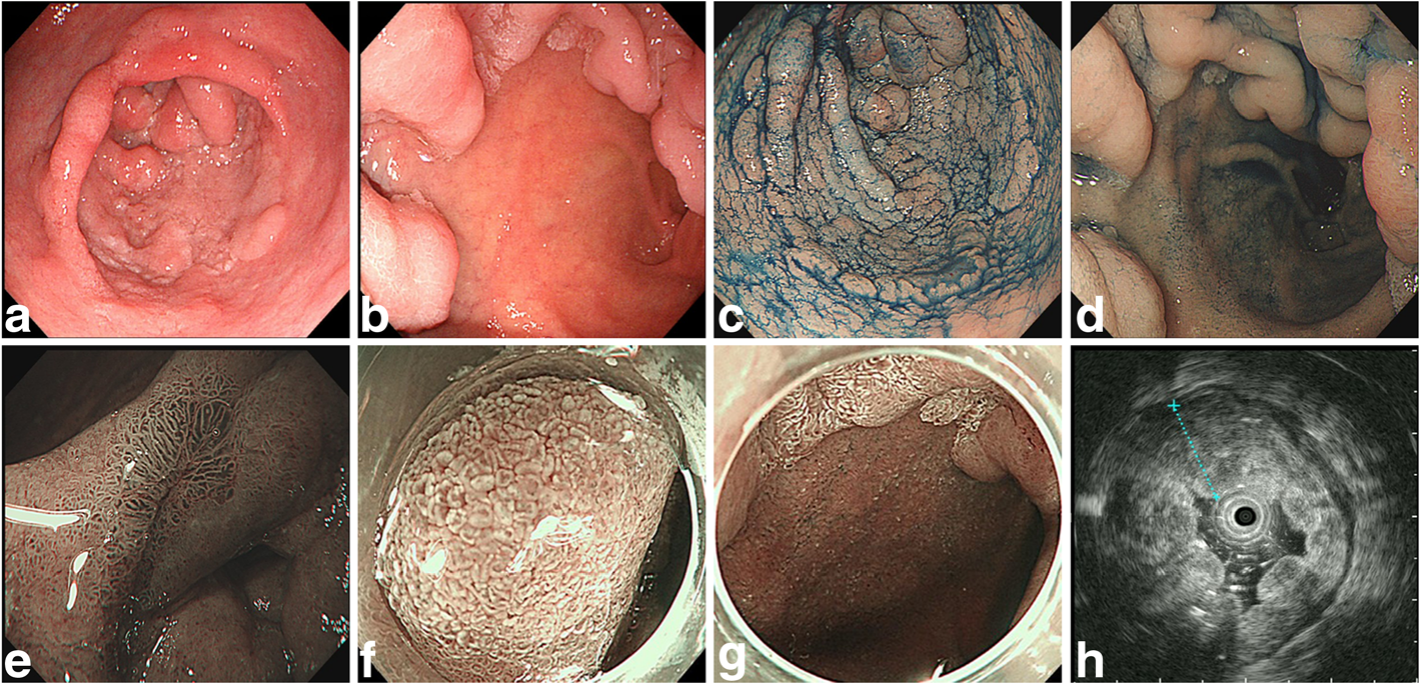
White light endoscopy showed a gastric laterally spreading tumour of the distal stomach of the granular nodular-mixed type, approximately 6 –cm in size, located at the antrum and pyloric region of the stomach; a 0-IIa + 0-IIC and 0-I lesion (a), along with a polypoid duodenal bulb lesion (b). Chromoendoscopy using indigo showed clear margins of the distal stomach (c) and duodenal bulb lesion (d). Narrow-band imaging revealed an irregular pit pattern of IIIL to IV and increased vascularization in the surrounding mucosa (e). A white opaque substance with oedematous villi is clearly seen in the antrum near the pyloric region (f), and duodenal bulb (g) indicated early gastric cancer. Endoscopic ultrasound showed a thickening of the mucosal layer of approximately 12 mm (h)
